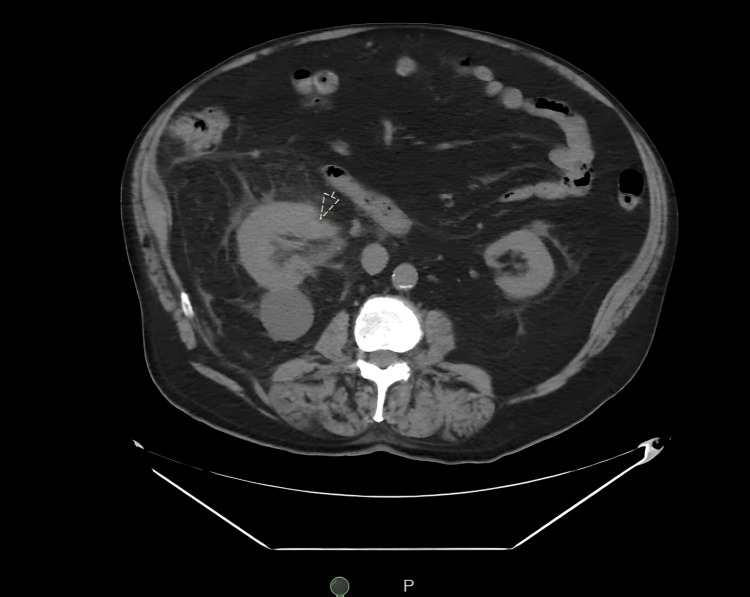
CT Abdomen/Pelvis Image Showing the Right Renal Mass
